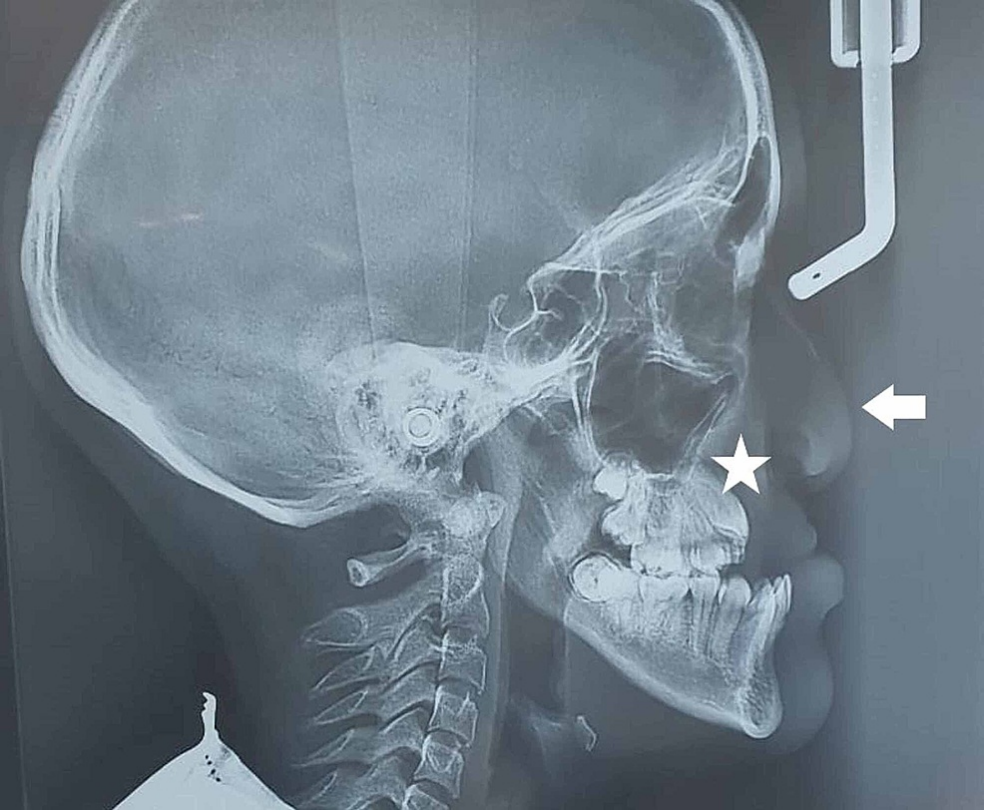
Lateral cephalic X-ray Mandibular prognathism was observed at the expense of maxillary hypoplasia (star). In soft tissues, there was drooping nasal tip (arrow); however, an unobstructed airway and preserved diameters were observed
